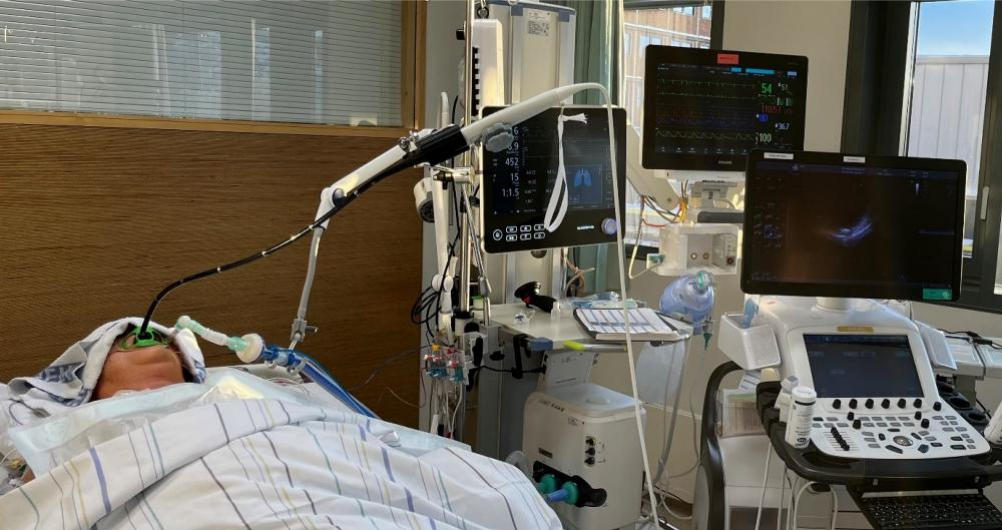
Continuous monitoring using hands-free transesophageal echocardiography. This photograph shows the transesophageal probe in a probe holder for hands-free image acquisition. The patient provided consent before being photographed.
